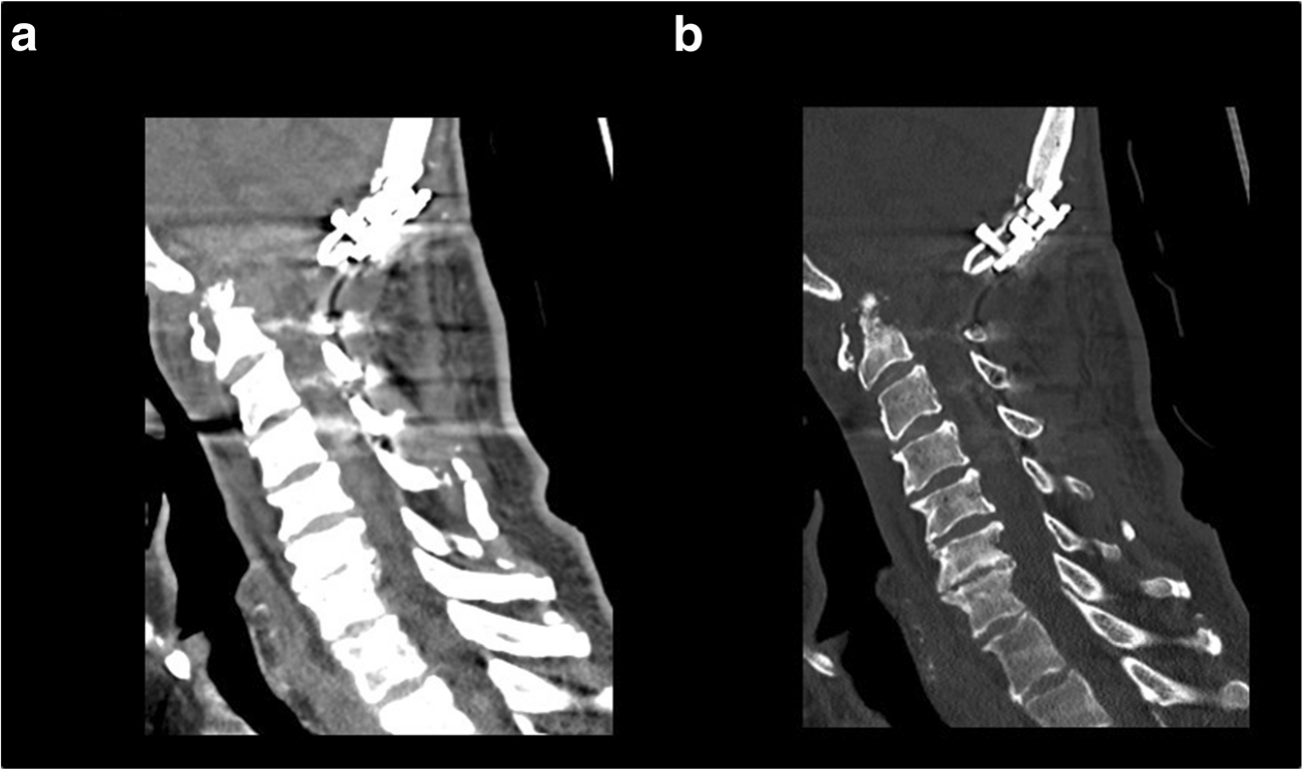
Sagittal CT scan of the cervical spine (a soft tissue window; b bone window) revealed no postinfectional changes of the OP without further destruction of the OP and revealed no signs of screw loosening
